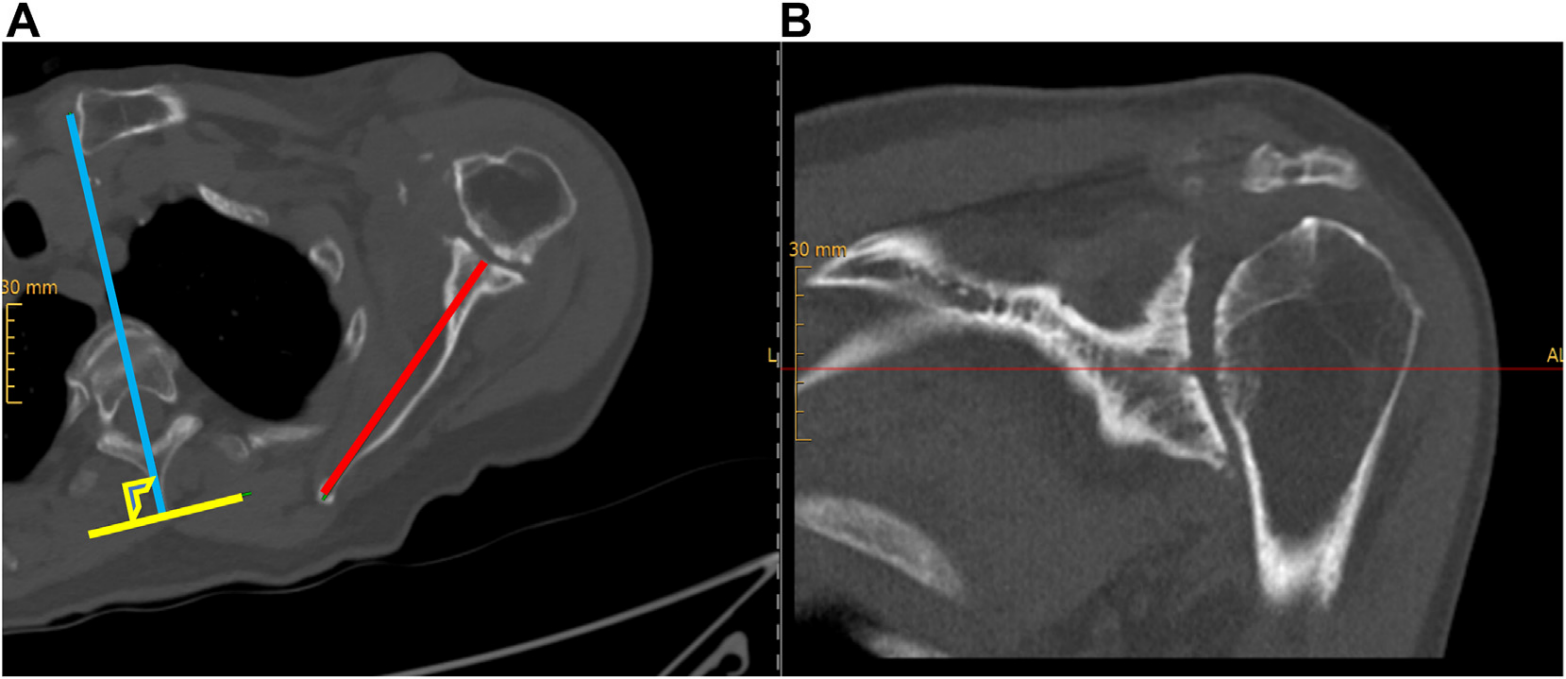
A computed tomography of a left shoulder in the axial (A) and coronal plane (B). The deepest point of the glenoid in both axial and coronal plane was defined. The *blue line* determines the parallel line to the spinal process, defining the axis of the vertebra. The *yellow line* shows the perpendicular line to the vertebral axis. The *red line* represents the line from the deepest point of the glenoid to the medial root of the scapula spine in the axial plane. The internal rotation of the scapula was then measured as the angle between the *yellow line* and the *red line*.
